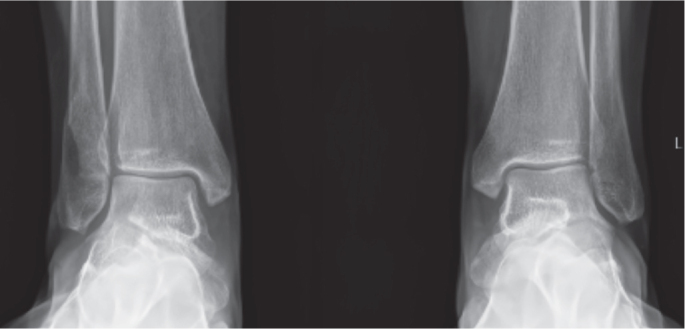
Weightbearing radiographs demonstrating symmetric ankle mortises in the injured and non-injured ankles.
